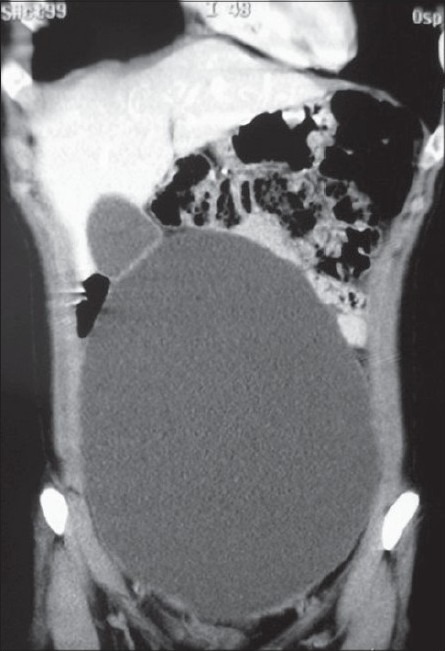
CT scan image of the large cyst
